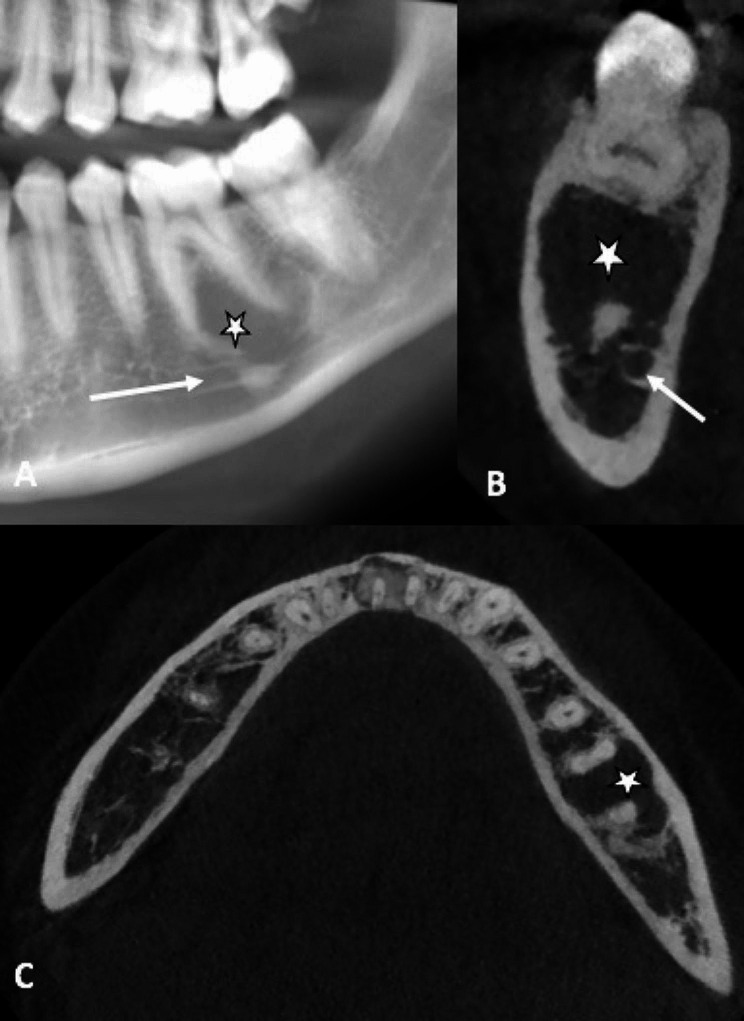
CBCT assessment. A. Reconstructed panoramic view. B. Orthoradial slice of mandible through tooth 36. C. Axial slice. Legend: These views show radiolucent lacuna surrounding roots of tooth #36 (white star*) (white arrow shows mandibular canal)
